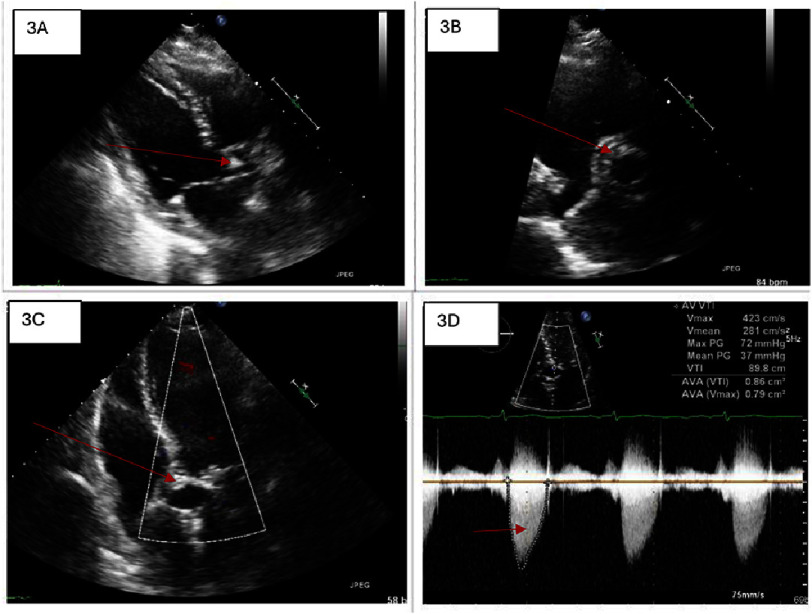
Transthoracic echocardiogram demonstrates a calcified and narrowed aortic valve as depicted by red arrows in 3A (Parasternal long axis), 3B (Parasternal short axis), and 3C (Apical five chamber view). The valve area was measured at 0.8 cm^2^ with a dimensionless index of 0.33 (Figure 3D).
